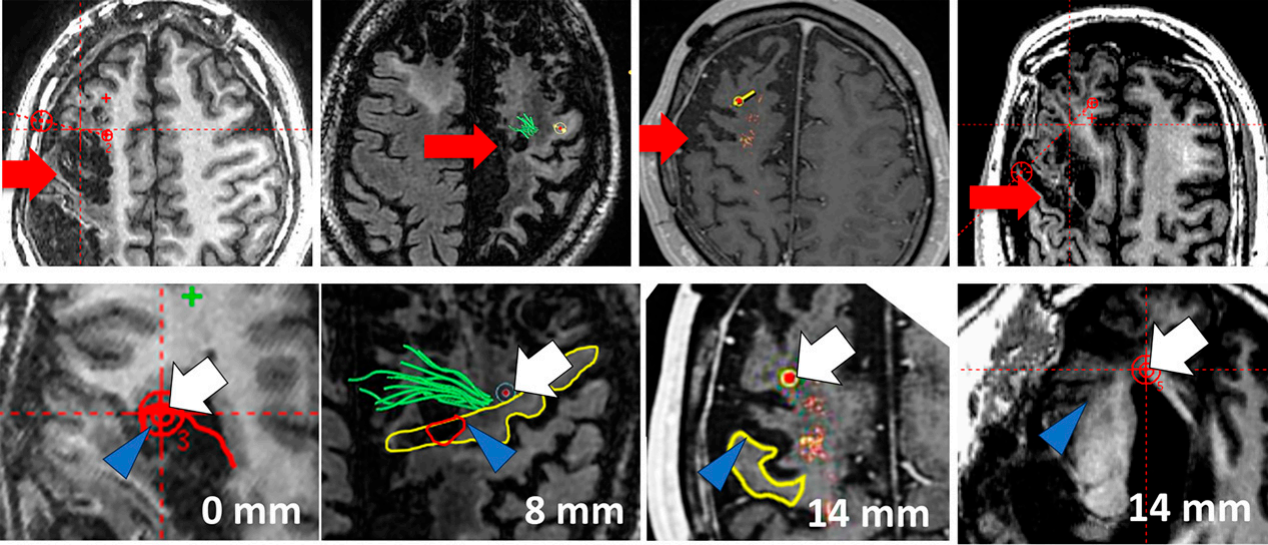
Representative figures of the patients with motor cortex damage. (Upper row) MRI axial image of the damaged area, where the red arrow indicates the actual damaged site. (Lower row) Prove eye views of the closest point between cell transplantation and the damaged area. The white arrow indicates the transplanted site, and the blue arrow indicates the closest point of the damaged area. The yellow area indicates the motor cortex, and the red circle indicates the responsible damaged area. MRI, magnetic resonance imaging.
